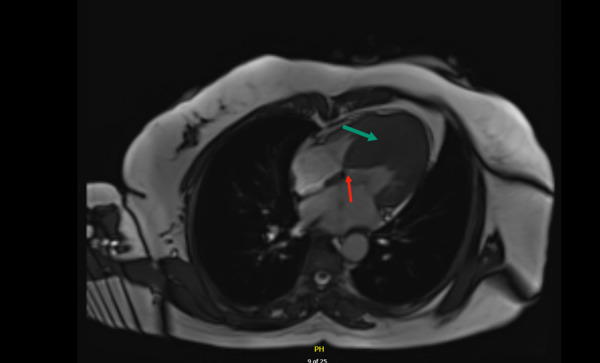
FIgure 3. Magnetic Resonance Imaging Cranial View 4 Chamber Systolic phase shows obliteration of left ventricular cavity during Systole (green arrow), and chordae systolic anterior motion causing Left Ventricular Outflow tract obstruction (red arrow).
